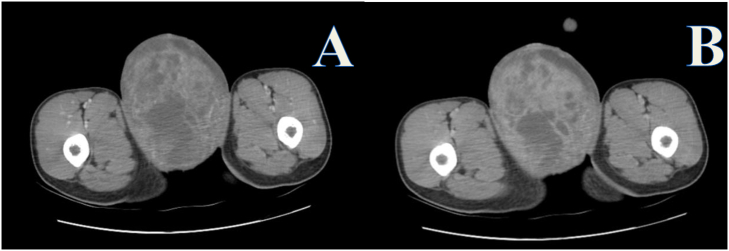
Contrast enhanced CT scan images with arterial (A) and portal venous (B) phases showing an heterogeneously enhancing huge scrotal mass with areas of necrosis.
